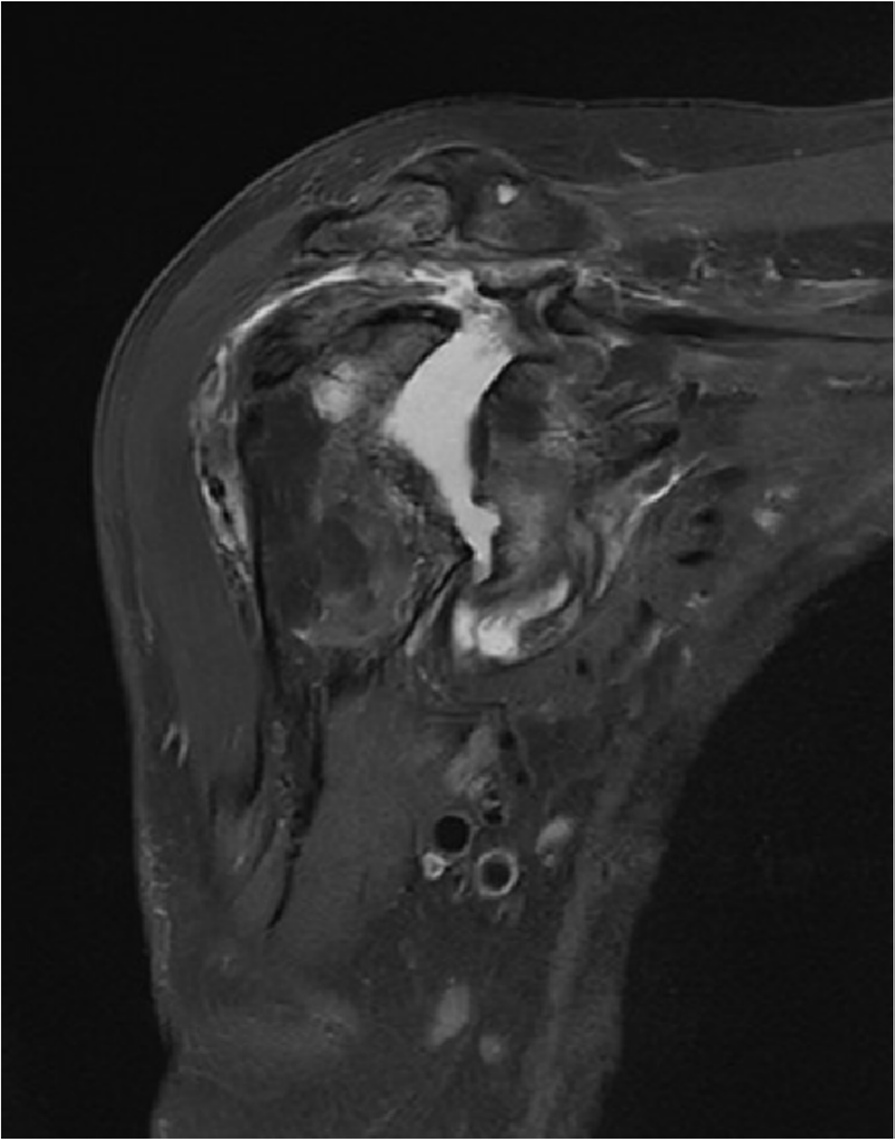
Coronal MRI of the right shoulder joint (fat-suppression sequence)
